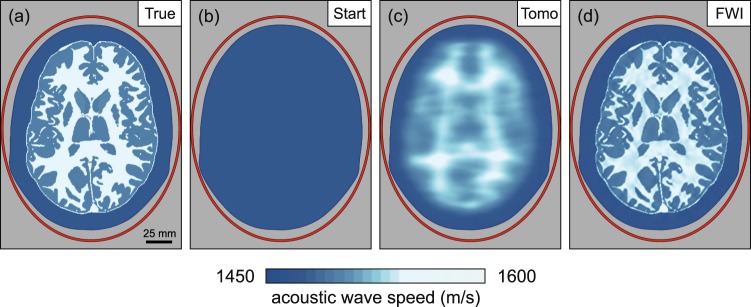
Inversion of data from a brain outside the skull. a A two-dimensional model of acoustic wave speed in the naked brain without the skull. The red ellipse shows the transducer positions; the grey region is masked and held fixed during the inversions. b Homogeneous model used to begin inversion. c Result of ultrasound computer tomography. The resultant model is accurate but has poor spatial resolution. d Result of ultrasound full-waveform inversion. The resultant model is now both accurate and spatially well resolved.
